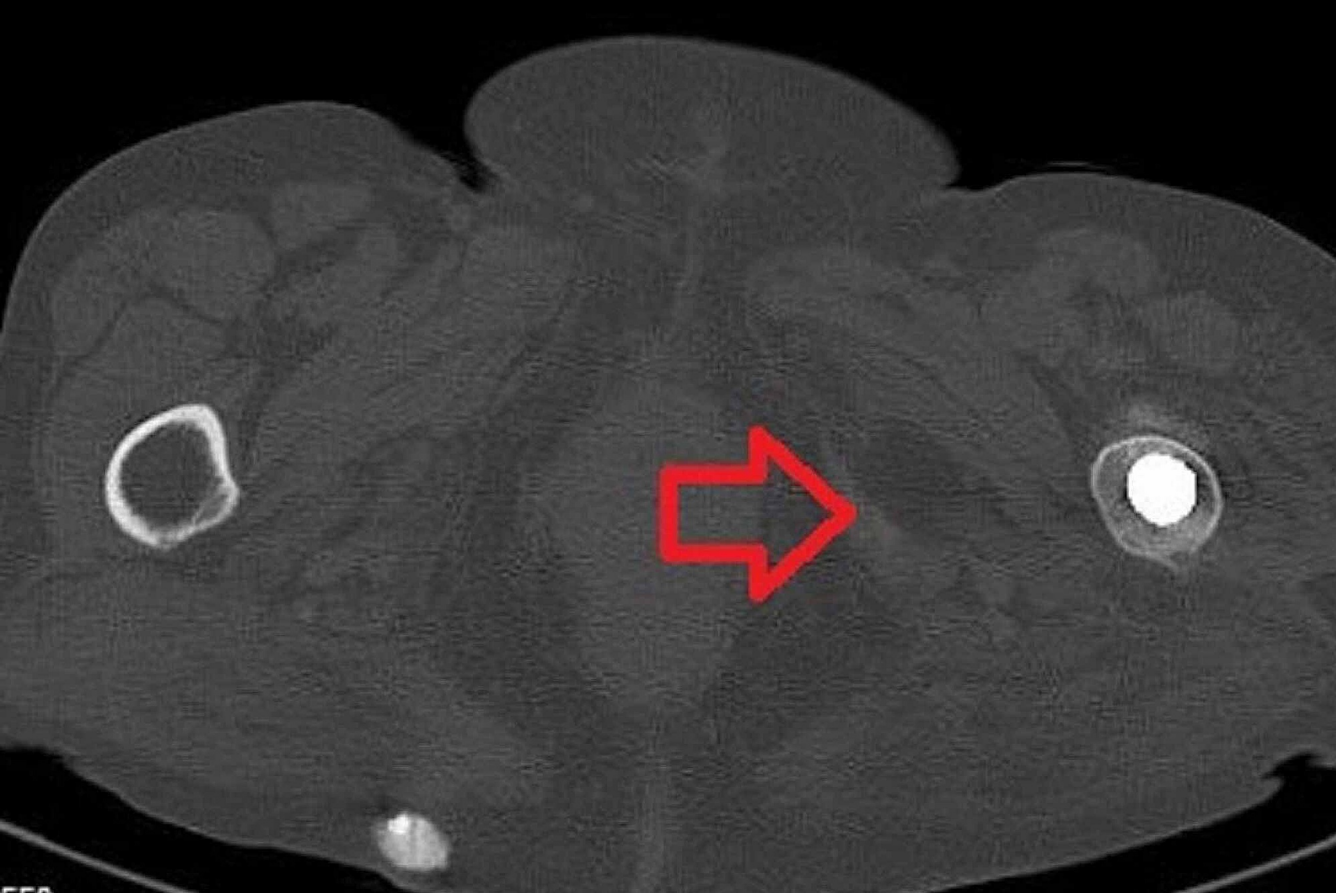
Axial computed tomography image of the affected hip joint The red arrow indicates the cyst formation beneath the adductors muscles.
